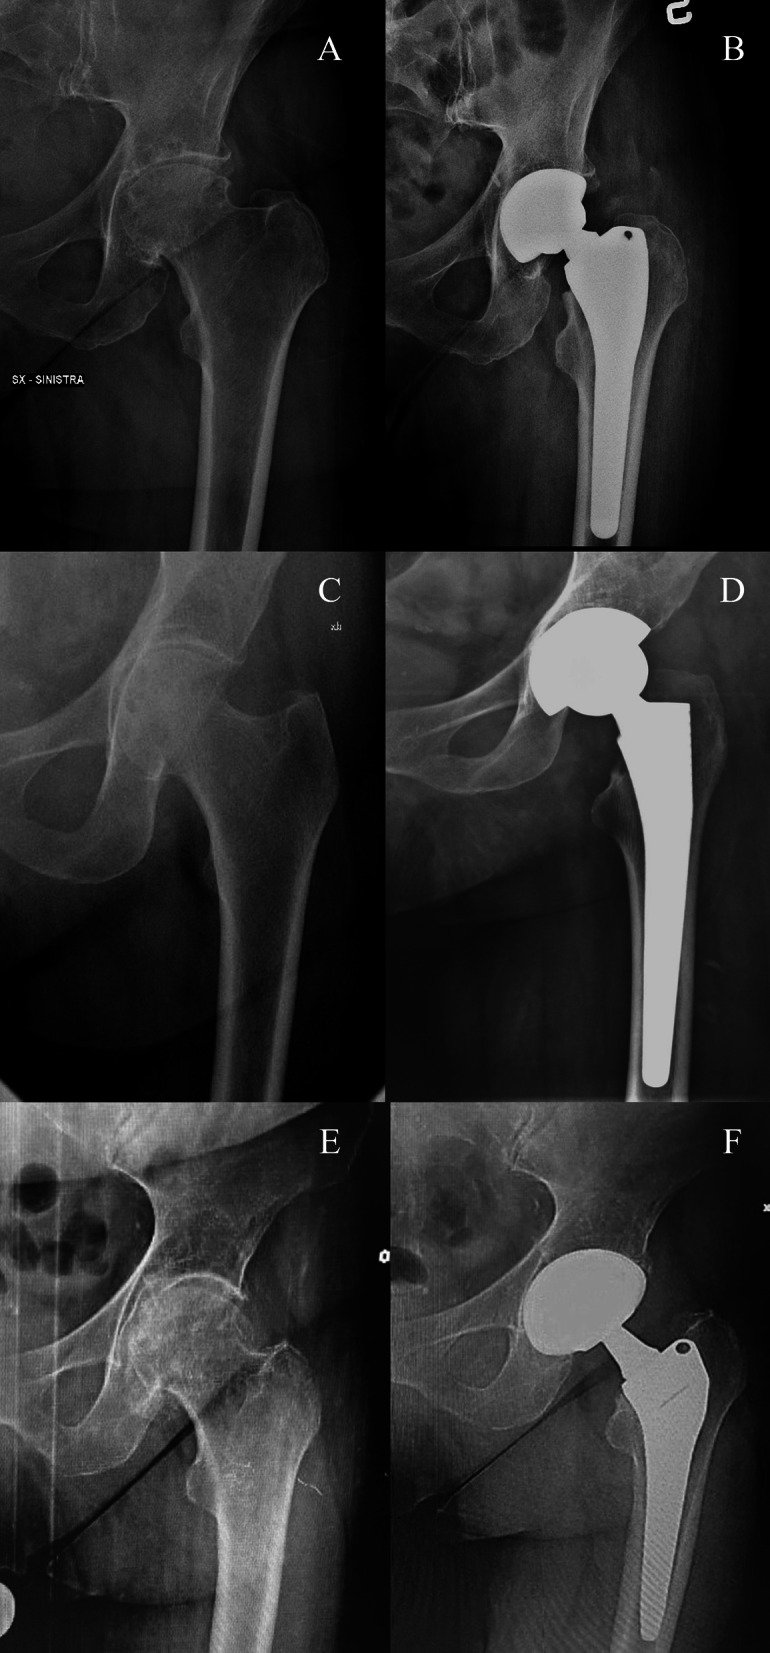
Preoperative (A, C, E) and postoperative (B, D, F) pelvis X-rays of three clinical cases, one for each stem with the DT version: Apta (B), Hydra (D), and Recta (F)
